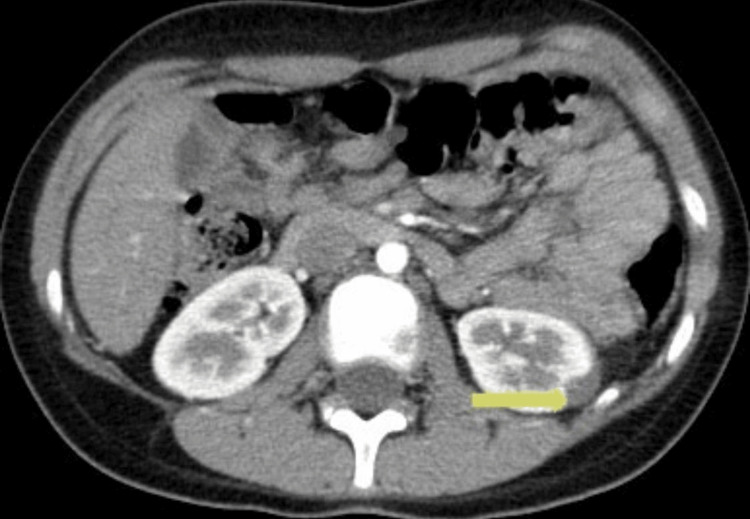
CT scan showing a reduction of the renal collection to 0.8 cm after three months of antibiotics treatment (yellow arrow).
